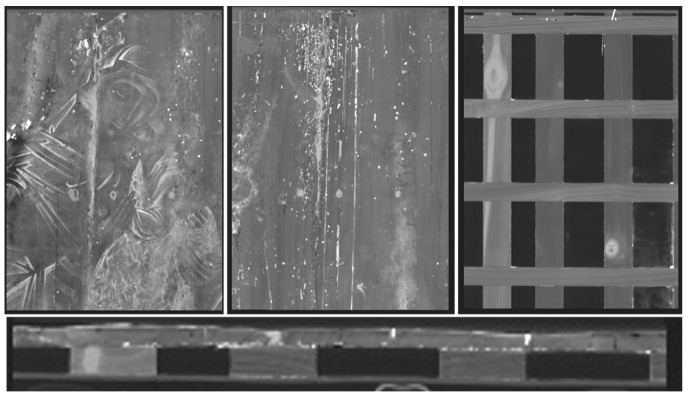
CT scan. (Top from left to right) front of the Icon, towards the Icon, the parking area; (down) the lower and upper section.
